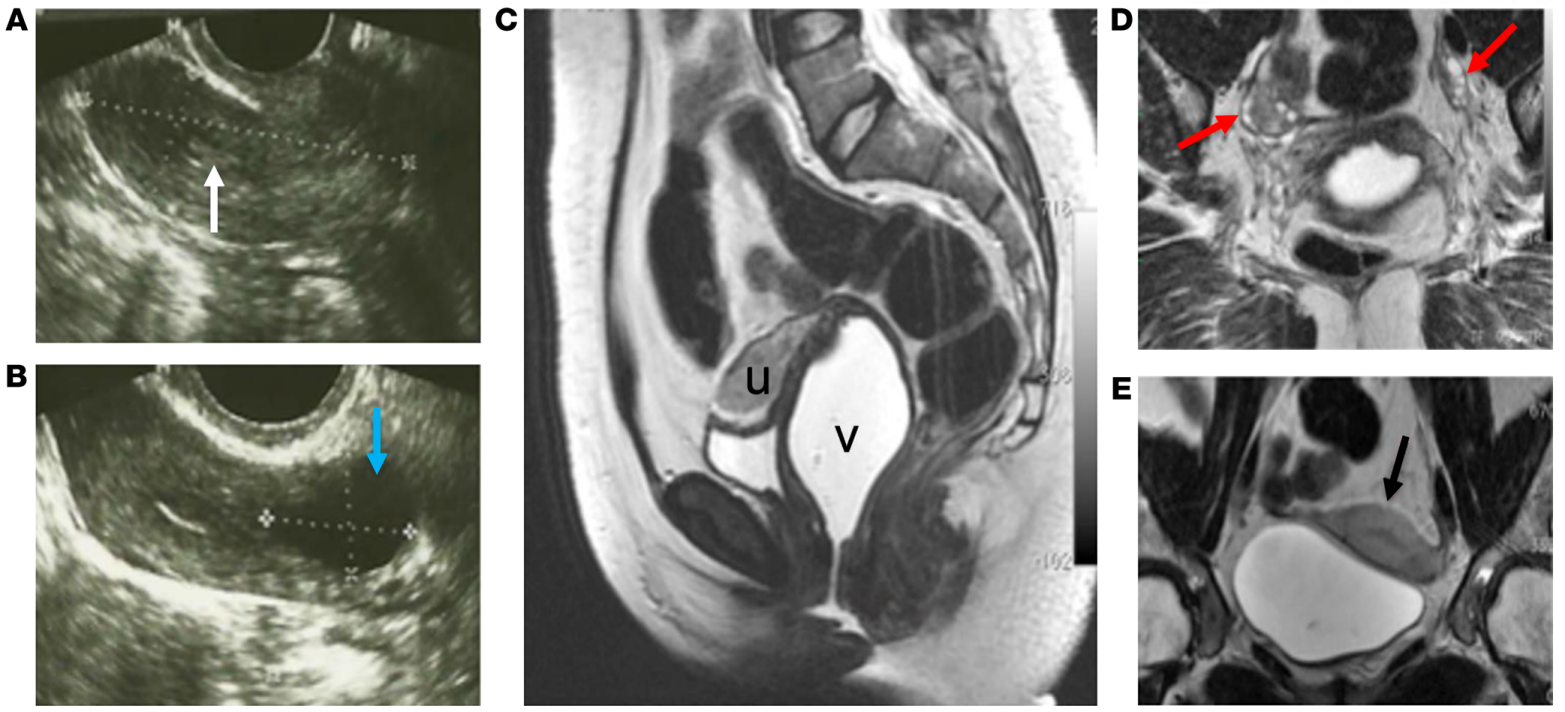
FRT imaging features of a 29-year-old woman (proband) with primary amenorrhea and a developmental anomaly of the reproductive tract. (A and B) Transvaginal ultrasonographic imaging showing (A) a hypoplastic uterus (dotted line) with a very thin endometrium (white arrow), and (B) a left cornual cystic lesion (blue arrow); (C–E) Sagittal (C), oblique coronal (D), and coronal (E) T2-weighted fast-recovery fast spin-echo (FRFSE) MRI showing pelvic sections with vaginal distention using aqueous gel, demonstrating: (C) widening of the upper 2 thirds of the vagina (v) and an anteverted hypoplastic uterus (u), (D) normal appearing ovaries (red arrows), and (E) a hypoplastic uterus with thin endometrium (black arrow). The MRI of the proband was performed while off of hormonal treatment for more than 1 year. No information about cycle phase was available since the patient was amenorrheic.
